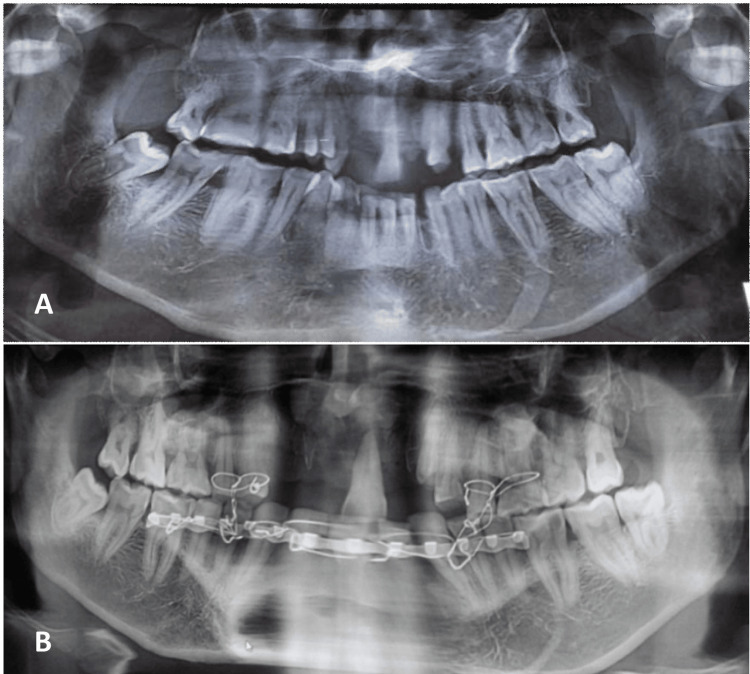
Orthopantomograph of mandibular parasymphysis fracture treated by closed reduction. (A) Pre-treatment. (B) Post-treatment.
